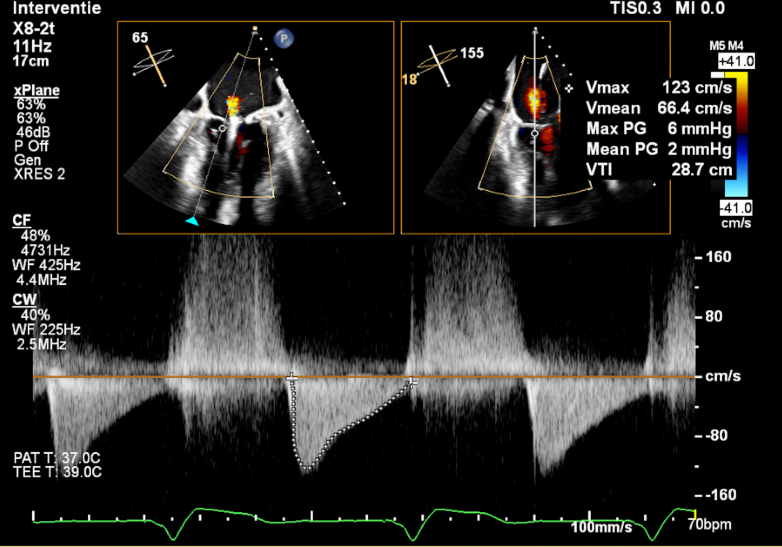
Assessment of Transmitral Postprocedural Gradient Transesophageal echocardiography showing normal transmitral gradient (2 mm Hg) after mitral transcatheter edge-to-edge repair.
